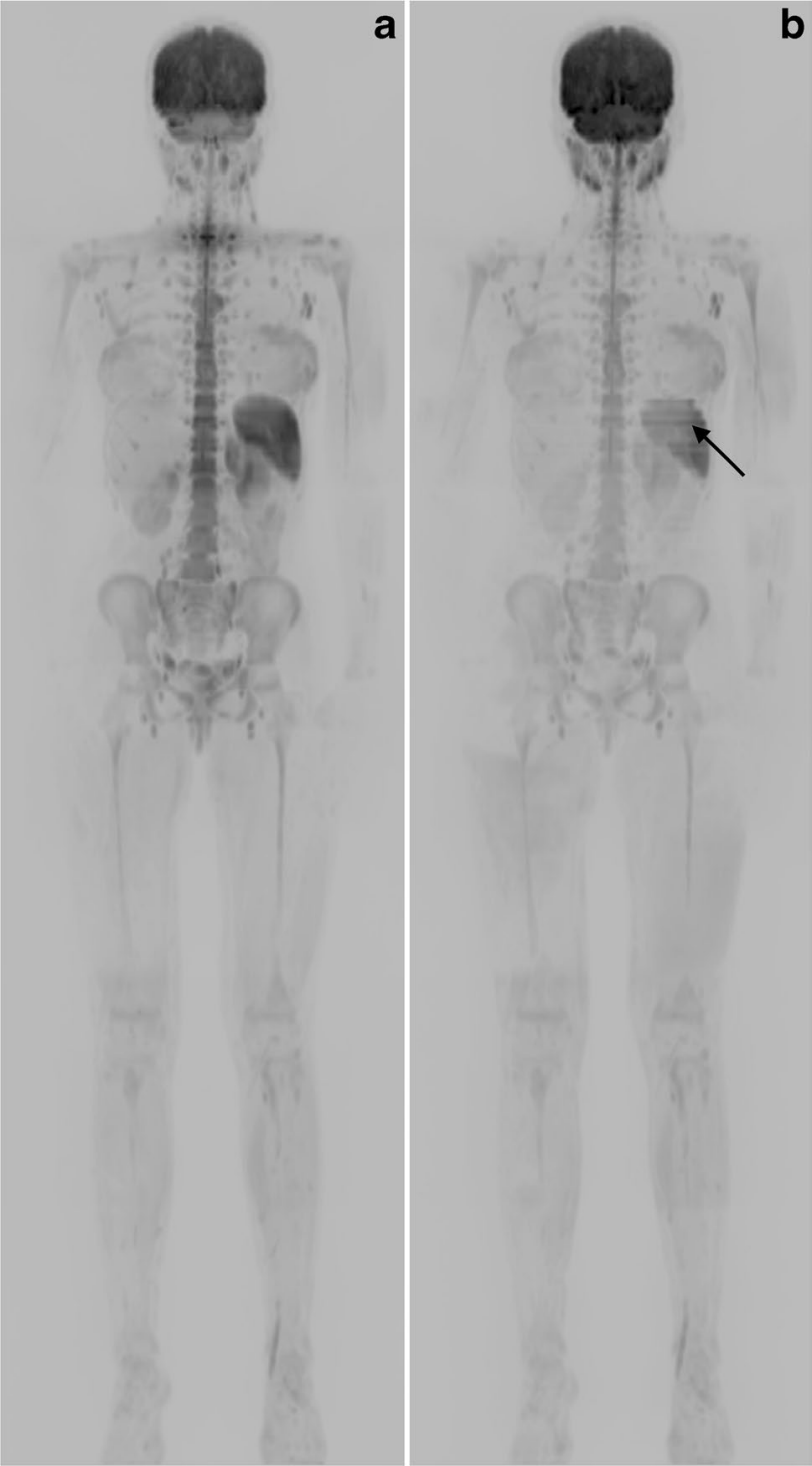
Comparison of the coronal 3-dimensional reconstructions for standard diffusion-weighted imaging (sDWI) (a) and simultaneous multi-slice diffusion-weighted imaging (SMS-DWI) (b) in a 15-year-old girl with cancer preposition syndrome. Both measurements with 6 stacks of DWI inline composed on the scanner. There are visible step formations in the SMS-DWI due to the different shimming algorithm without slice adjustments (black arrow). Overall, however, there is comparable quality with a homogeneous signal
